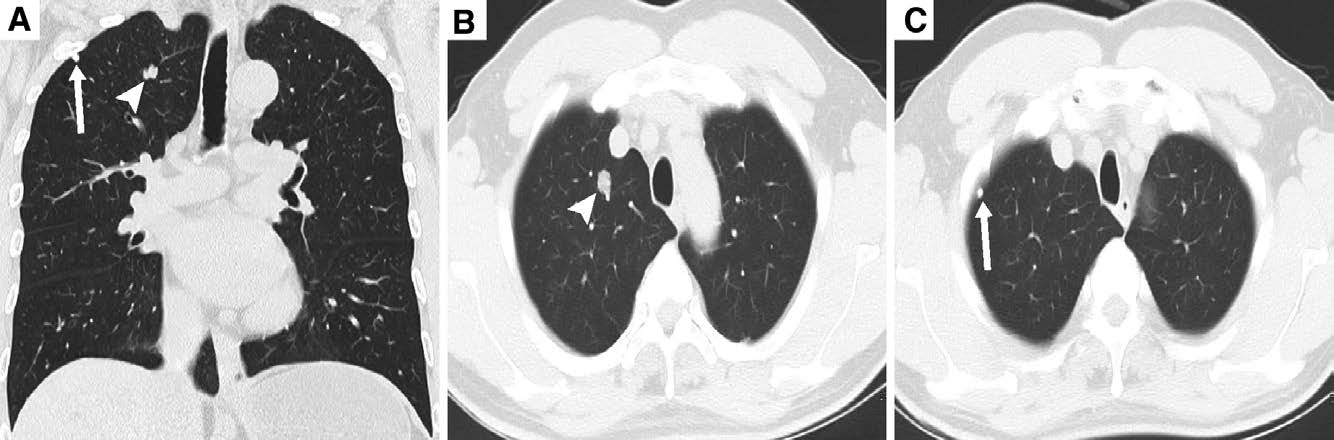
(A) Thoracic CT scan described 2 well-circumscribed pulmonary nodules in the right upper lobe. (B, C) These 2 lesions were located at the apex of the lung (white arrow-head) and on the surface of visceral pleura (white arrow) respectively.
